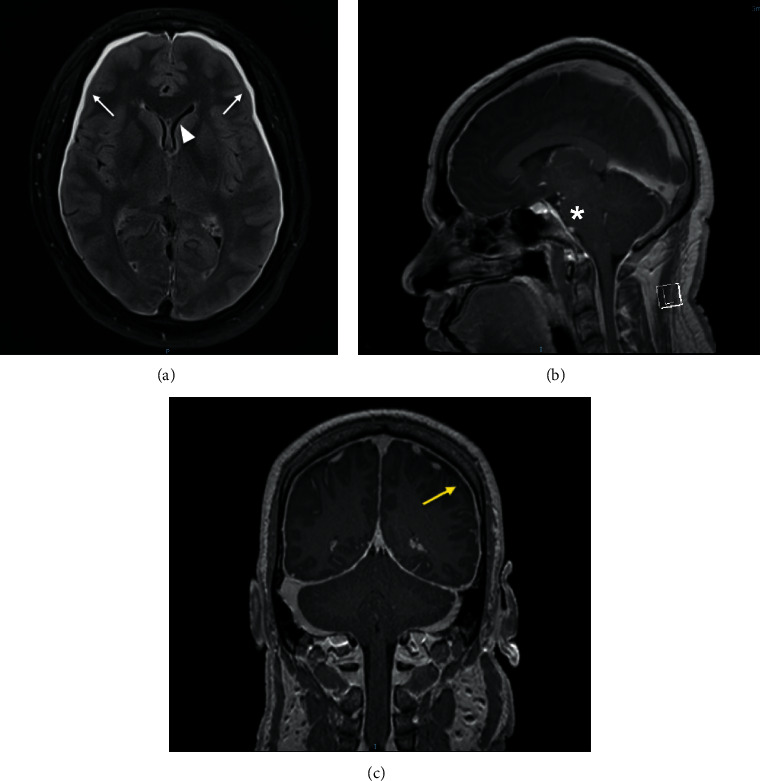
MRI of the brain showing features of intracranial hypotension. (a) FLAIR: bilateral subdural hygromas (white arrows) and slit-like ventricle (white arrowhead). (b, c) Post-contrast: reduced pre-pontine space (asterisk) and diffuse pachymeningeal enhancement (yellow arrow).
